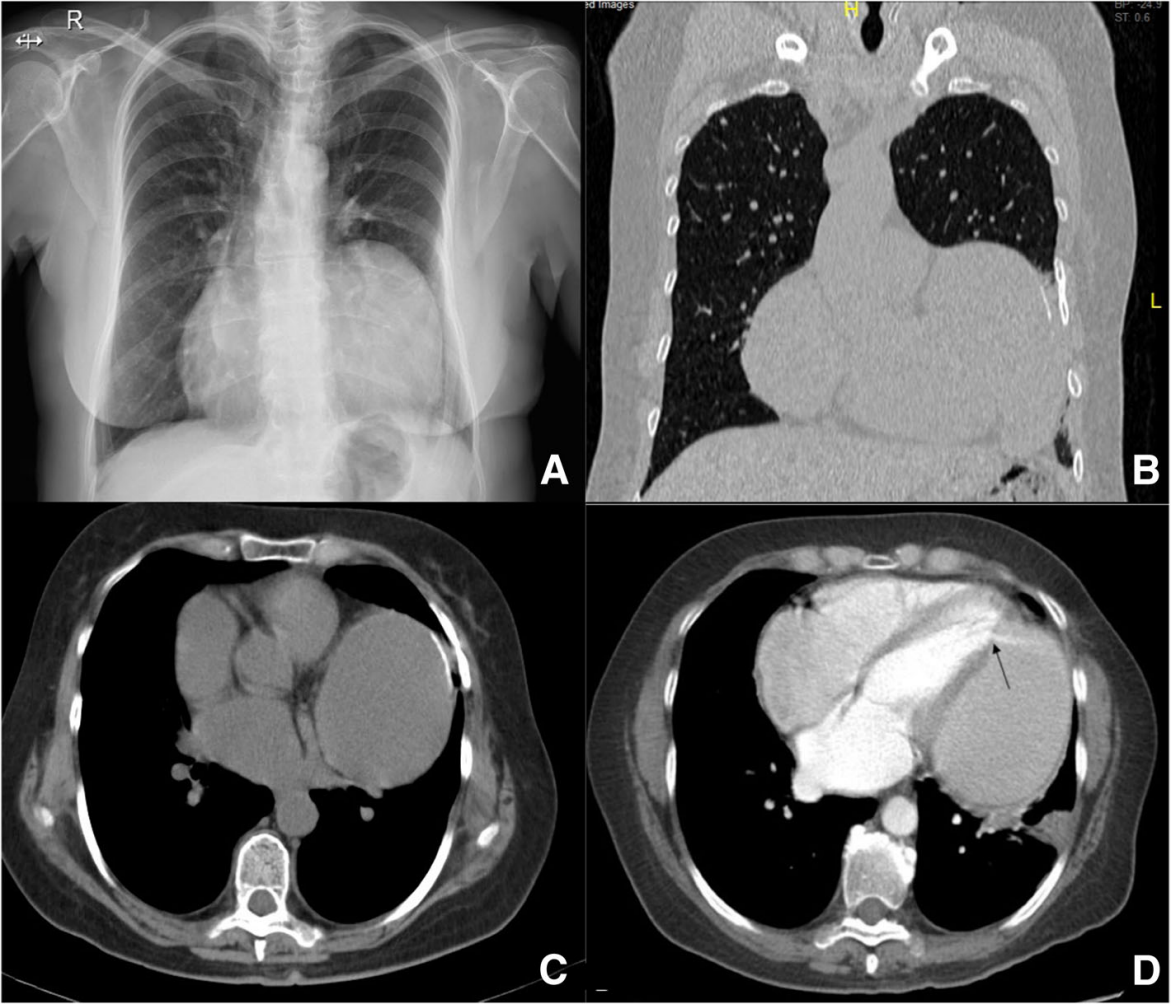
a Chest x-ray showing ballooning of the left cardiac silhouette, without pulmonary infiltrates or pleural effusion. b Sagittal scan of the left ventricular lesion. c Thoracic computed tomographic (CT) scan: appearance of the ventricular pseudoaneurysm with thin pericardial wall. d Thoracic CT-scan showing the leakage of contrast medium within the cavity of the ventricular pseudoaneurysm, and this corresponds exactly to the black arrow. *R* right side, *L* left side
